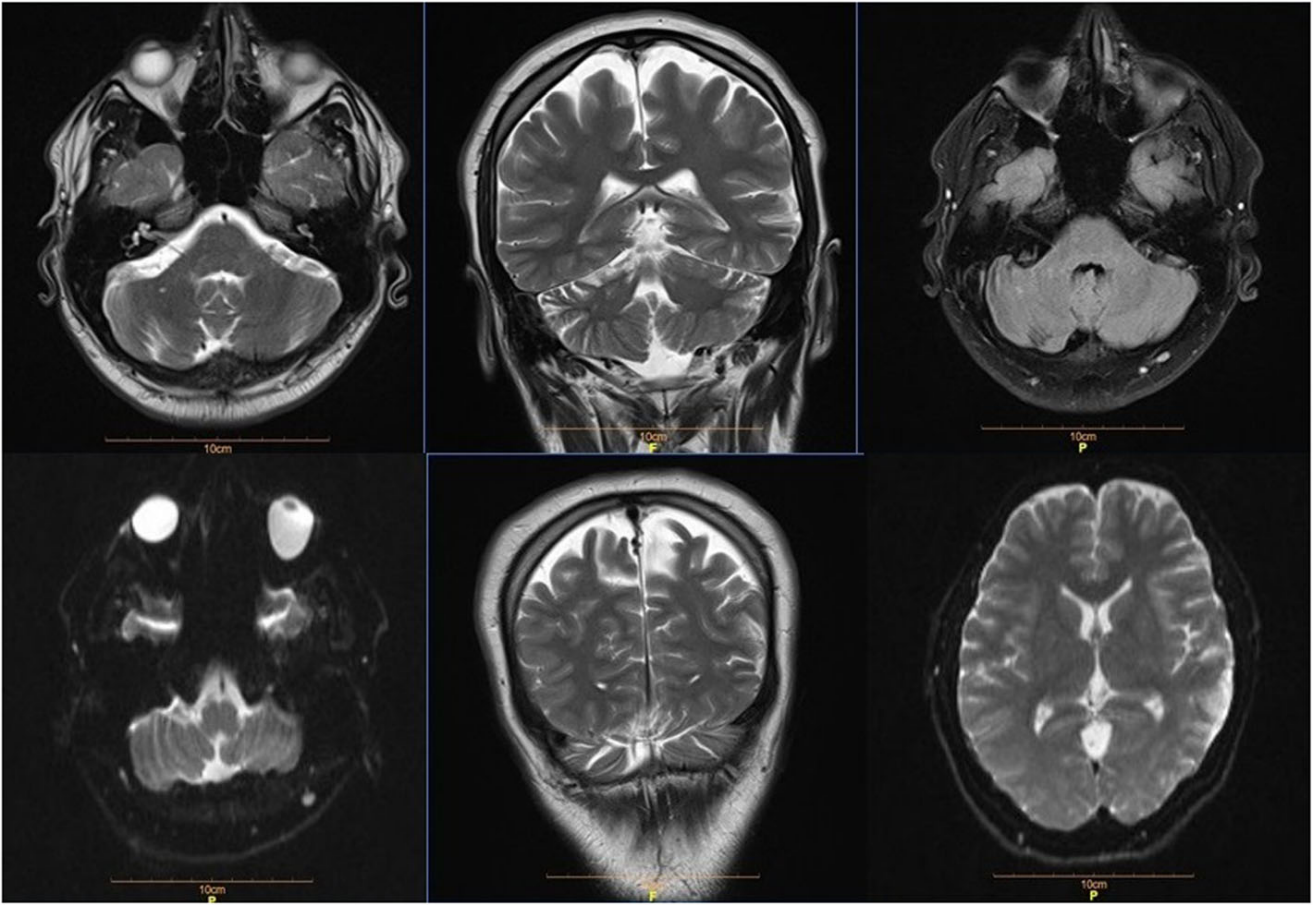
Brain MRI was done 3 months later that demonstrated relative improvement in prior ischemic lesions without the development of any new lesion
